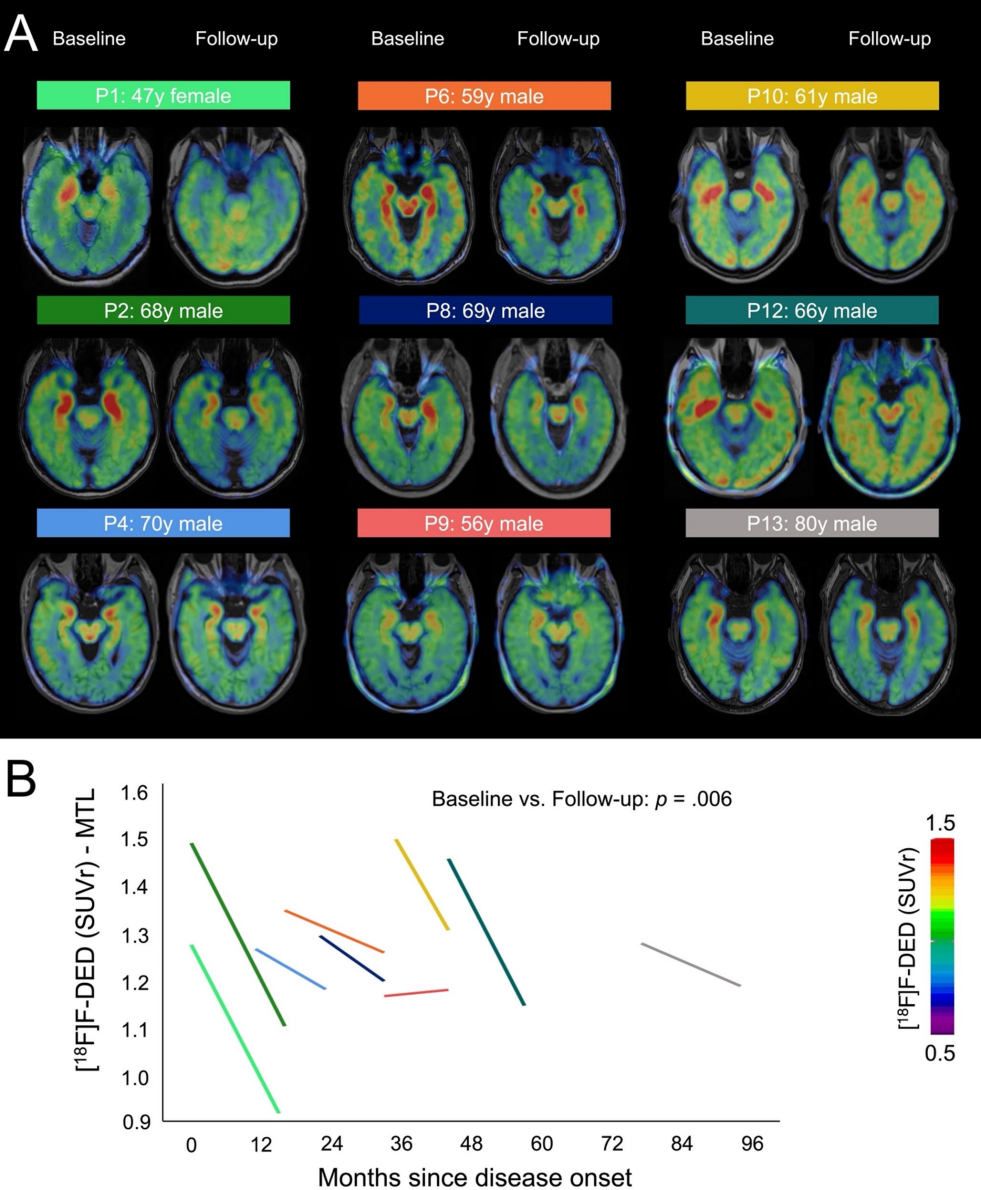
[^18^ F]F-DED PET allows monitoring of astrogliosis (A) Axial planes of [^18^F]F-DED PET images (SUVr, referenced to straight gyrus) fused with individual MRI (T1 weighted) of nine LGI1-AE patients comparing baseline to follow-up scan. (B) Quantitative [^18^F]F-DED PET SUVr (referenced to straight gyrus) of nine LGI1-AE patients comparing baseline and follow-up signals in the medial temporal lobe (MTL) (manual delineation of elevated regional signal at baseline). Two-sided paired t-test (*p* =.006)
